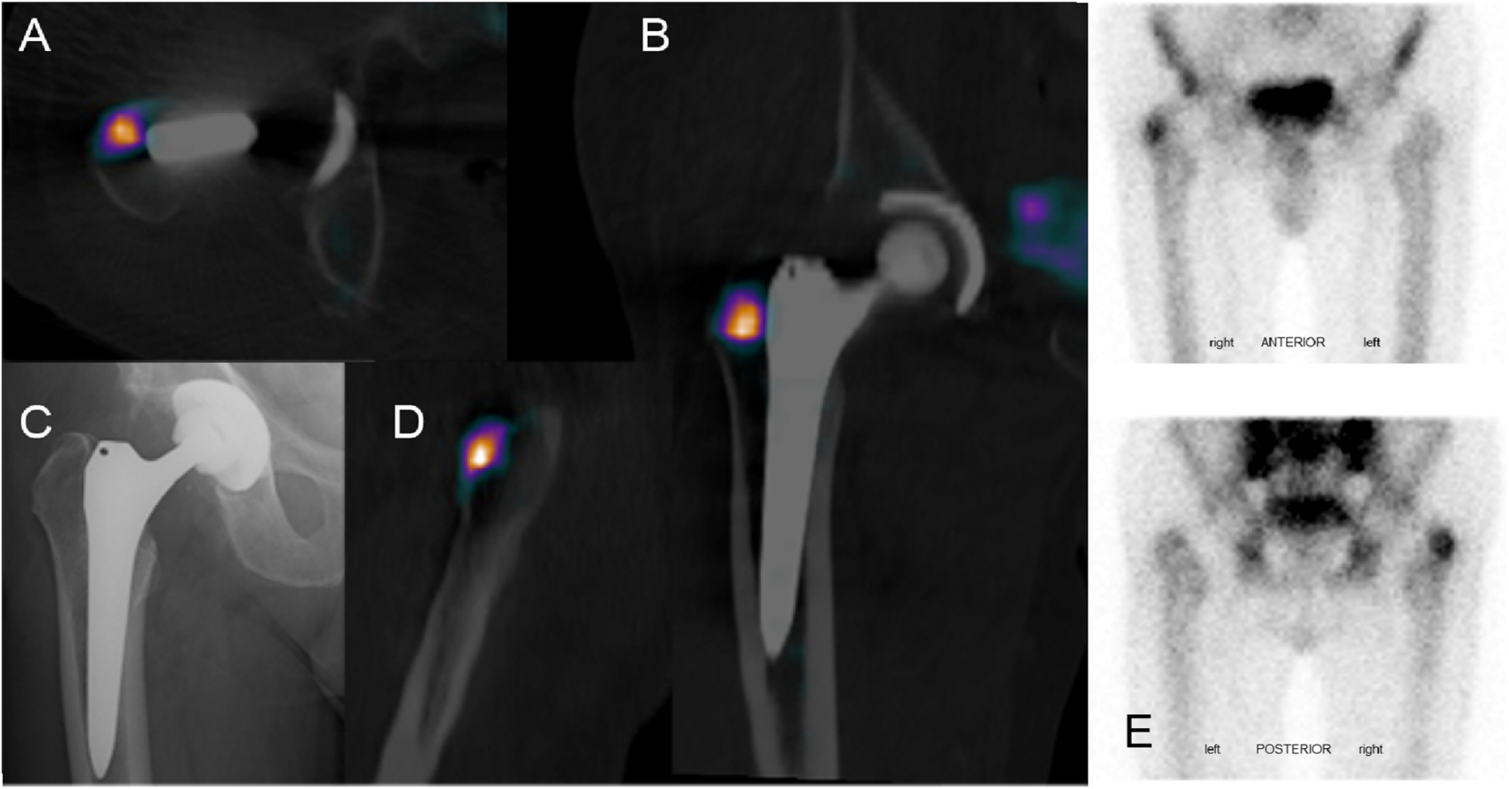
Example of physiological tracer uptake in THA. 77-year old male patient with a diaphyseal fixating stem of the right hip, suffering from pain in the right hip. C) shows the stem in varus position and a radiolucent line (stress shielding) in Gruen zone 1. A), B) and D) show increased bone metabolism in the greater trochanter. This represents physiological enhancement and is consistent with the grading F2,1. After further investigations a spine-related cause of the pain was diagnosed via MRI.
